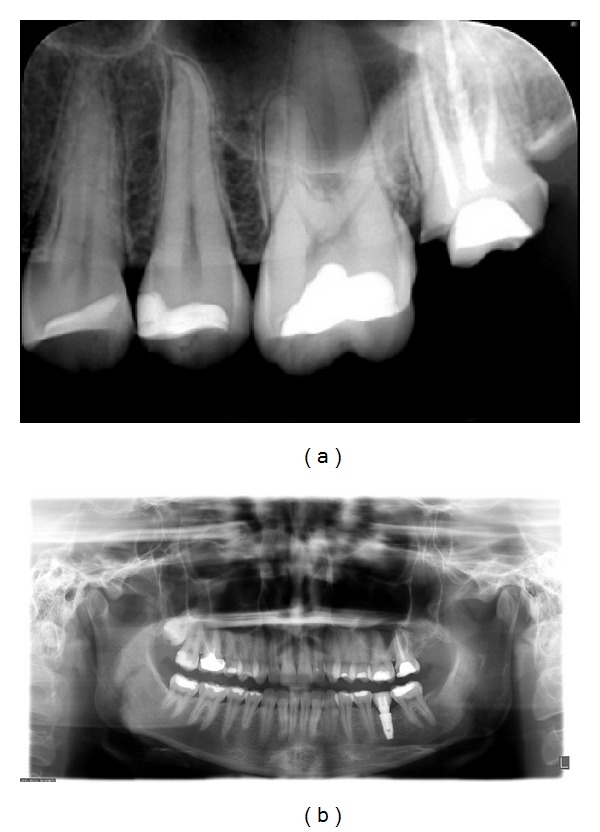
(a) and (b) Periapical and panoramic films show no evidence of lesion in the upper region of left second molar.
